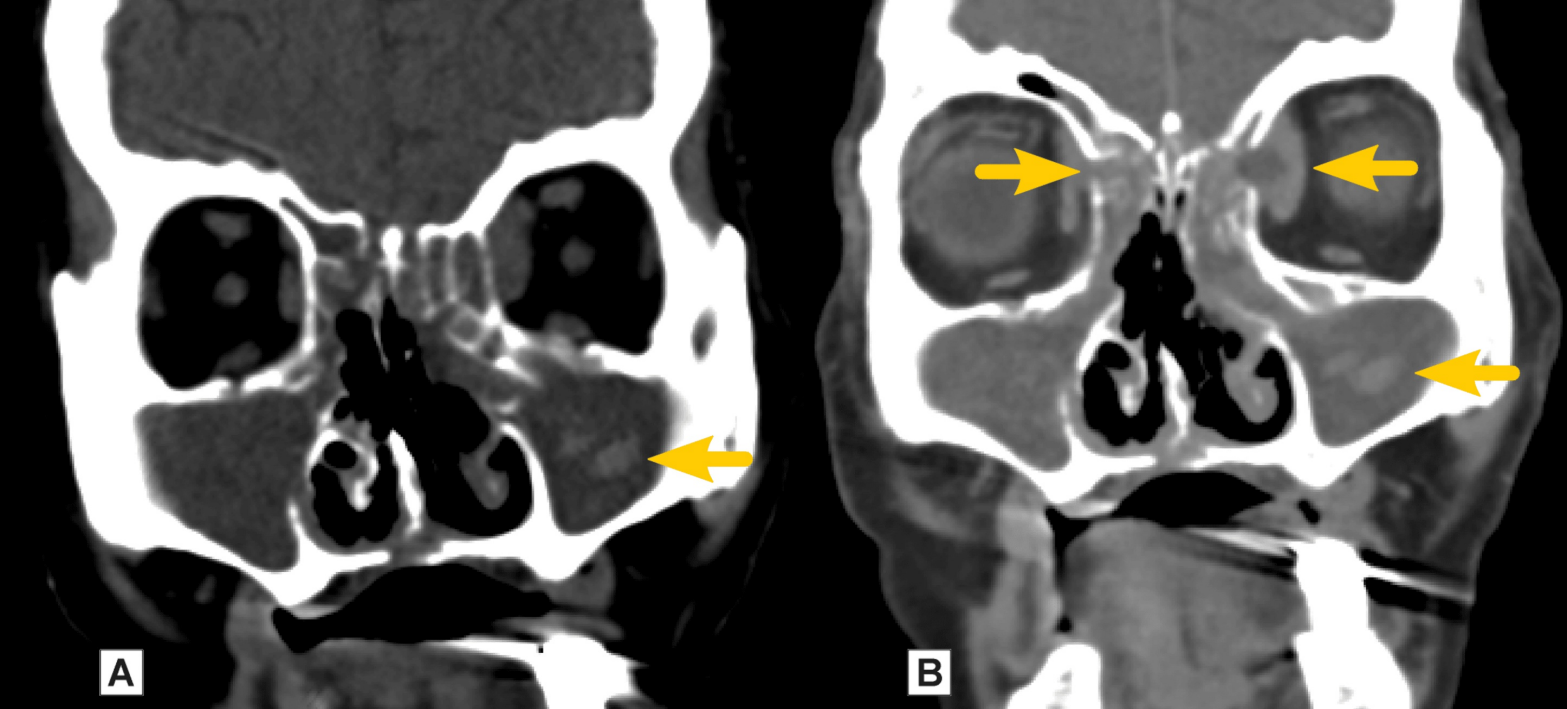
CT findings (Case 2) A: native CT in the coronal plane showing hyperdense regions in the maxillary sinuses (arrow); B: postcontrast CT showing no significant contrast enhancement of the regions and thinning and osteolysis of the medial wall of the right orbit with some fluid collection (arrow)
